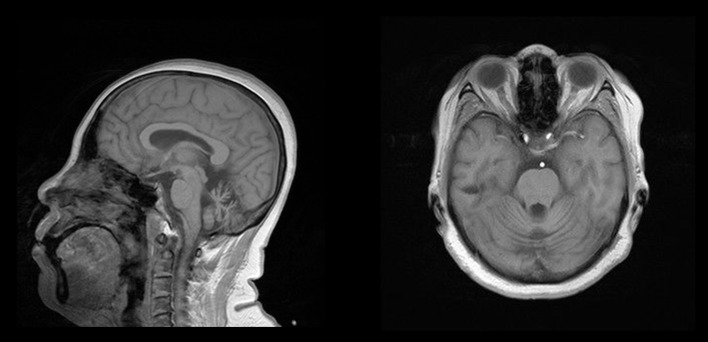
Brain MRI of the proband. Note the presence of obvious cerebellar atrophy.
